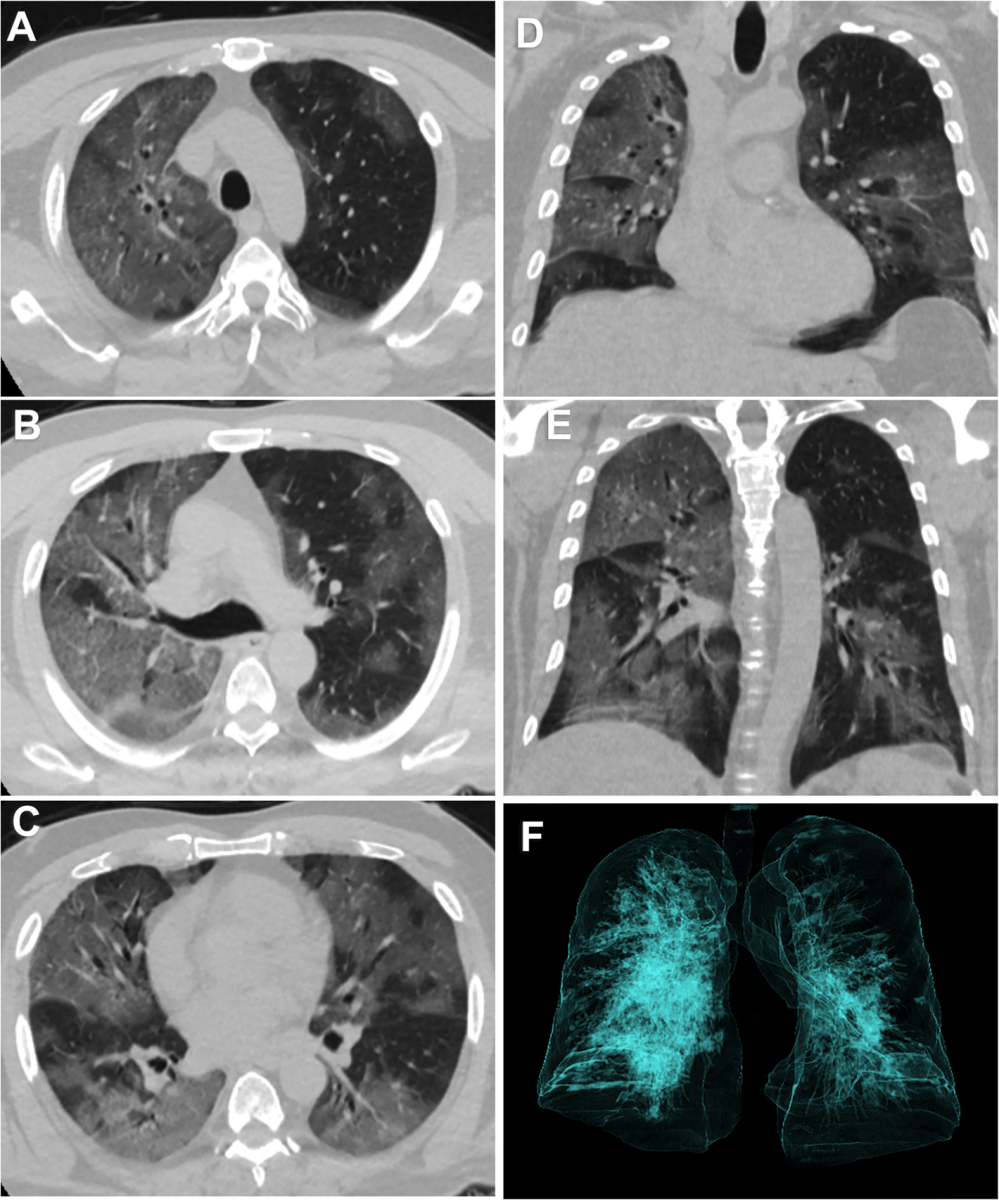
Non-contrast axial CT of the chest of a 58-year-old male patient with positive RT-PCR. Bilateral diffuse, more peripheral ground-glass opacities are seen with prominent vessels in the affected areas (a–e). 3D reconstruction volume rendering image shows pulmonary involvement as opaque non-transparent shadows (f). CT severity score is 16 points which corresponds to moderate involvement. The patient died on follow-up
